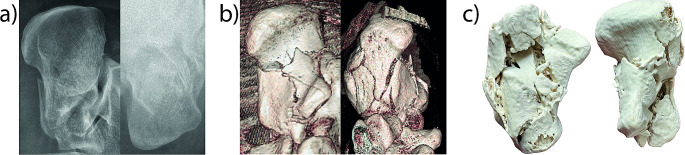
Radiograph, 3D reconstruction, and 3D print of a triple fracture of the calcaneus (Case 4)
